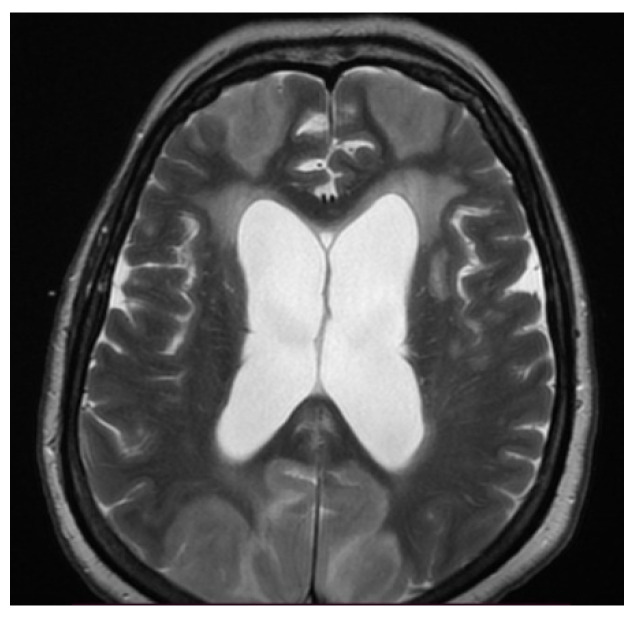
MRI axial T2-weighted sequence showed bilateral subcortical confluent hyperintense lesions with vasogenic edema in the frontal, parietal, and occipital lobes and secondary hydrocephalus.
